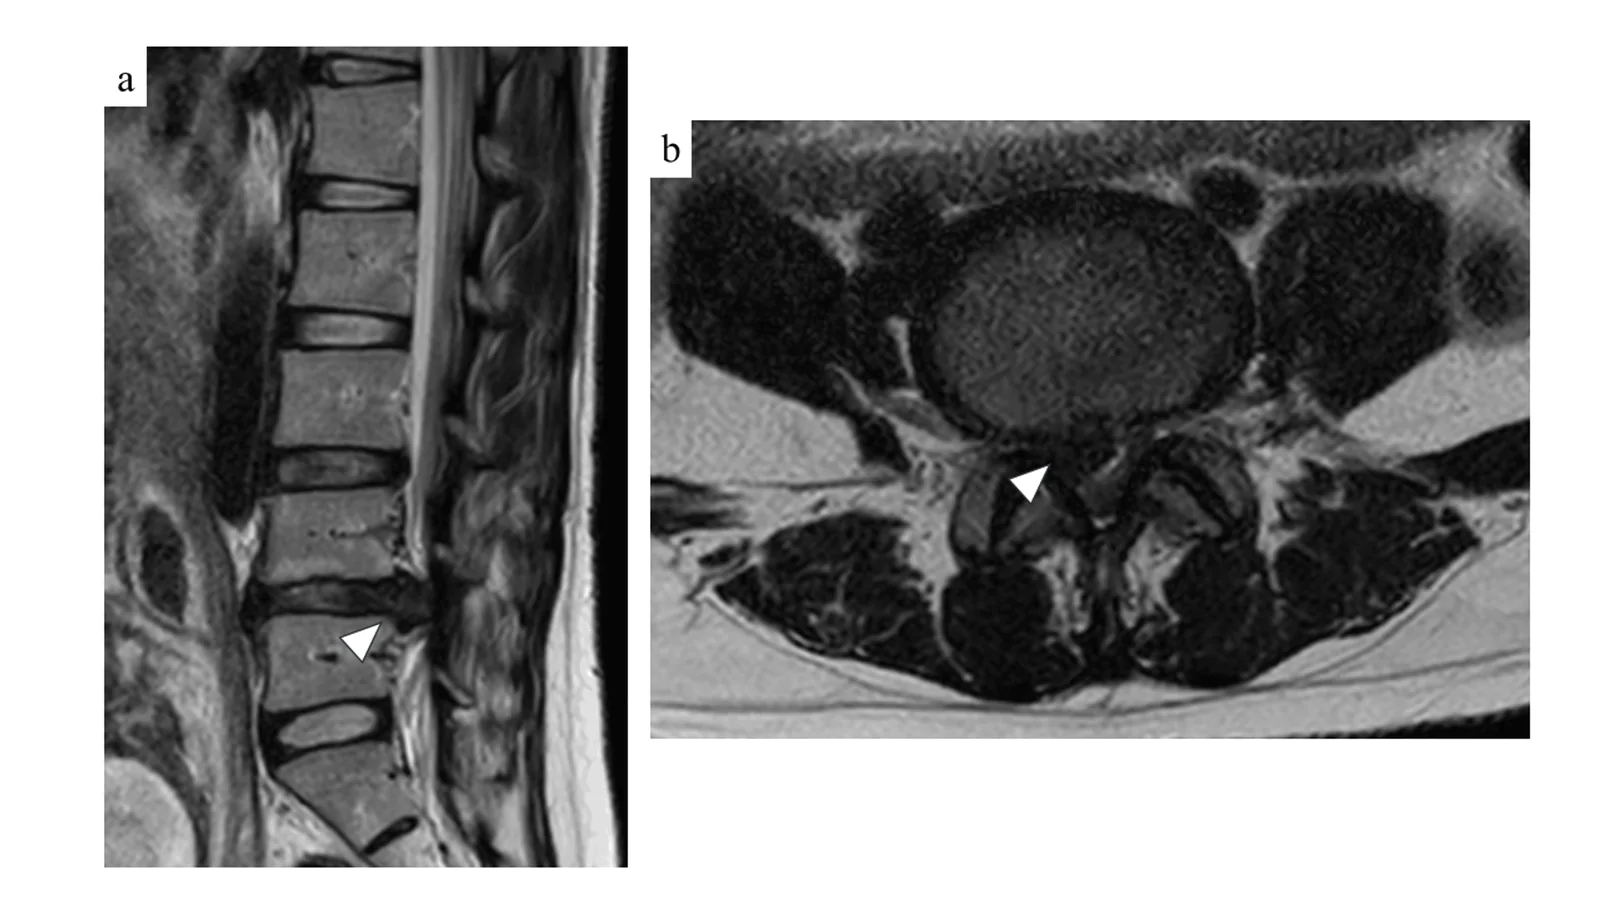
T2-weighted magnetic resonance images of the lumbar spine (a) Sagittal image showing a lumbar disc herniation at the L4–L5 level (arrowhead); (b) Axial image showing the herniated disc compressing the dural sac and adjacent nerve root at the same level (arrowhead).
